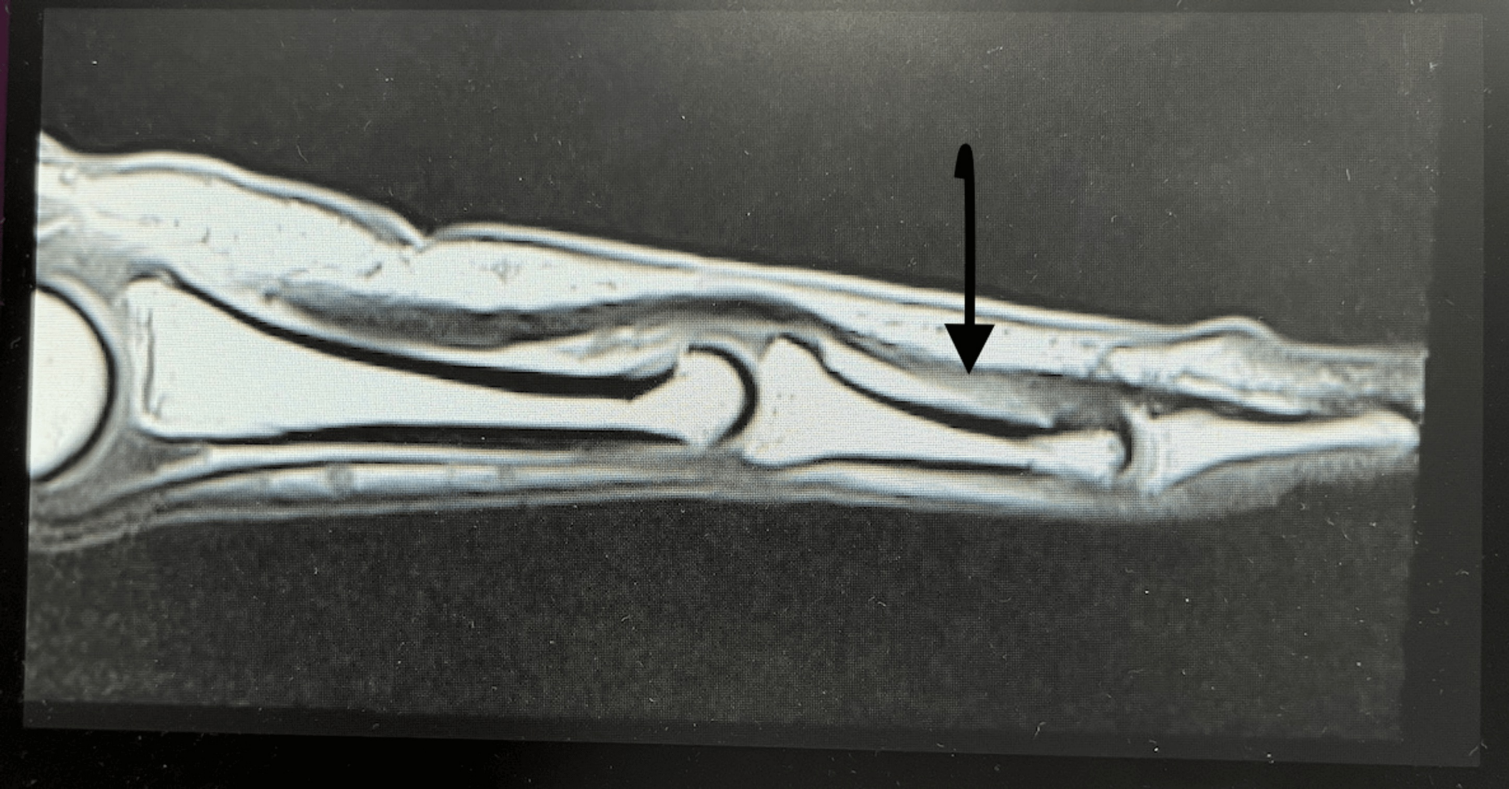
T1-weighted turbo spin echo sequence acquired in the sagittal plane of magnetic resonance imaging at WL 182 and slice thickness 2.5 mm, showing absence of FDPt in index finger Arrow shows the insertion of FDSt near the head of the middle phalanx, which is distal to its normal insertion. There is no FDPt. FDSt - flexor digitorum superficialis tendon; FDPt - flexor digitorum profundus tendon; WL - window level
